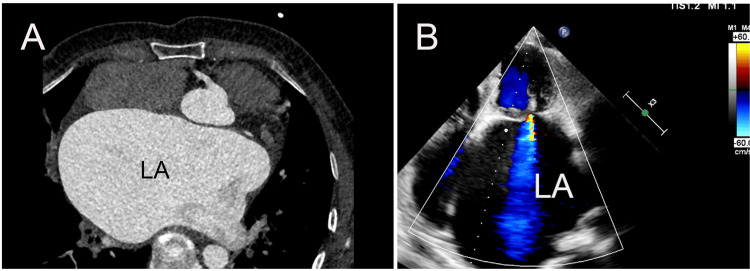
Cardiac CT and TTE showed a giant left atrium (LA). (A) Cardiac CT revealing a markedly enlarged LA compressing the adjacent cardiac chambers with a diameter of 75 mm. (B) TTE (apical four-chamber view) confirming the presence of a giant LA. CT: computed tomography; TTE: transthoracic echocardiography.
